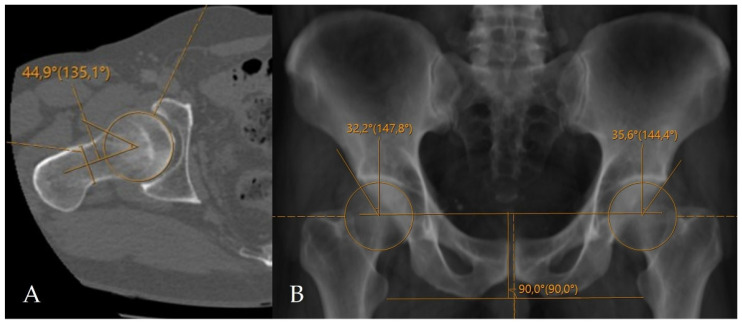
Application of conventional angular measurement methods on a radiology workstation. (A) Measurement of the alpha angle on axial oblique CT reformats. (B) Measurement of the LCEA on a CT-based virtual pelvic radiograph.
